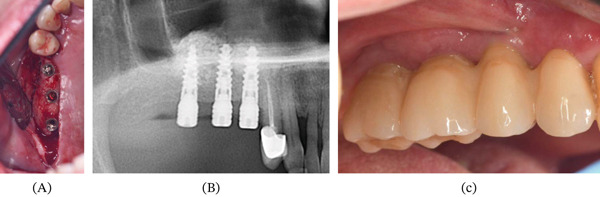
Implants placement 12 months after curettage surgery: (A) clinical view and (B) panoramic radiograph. (C) Final implant‐supported prosthetic rehabilitation at 30 months of follow‐up.
